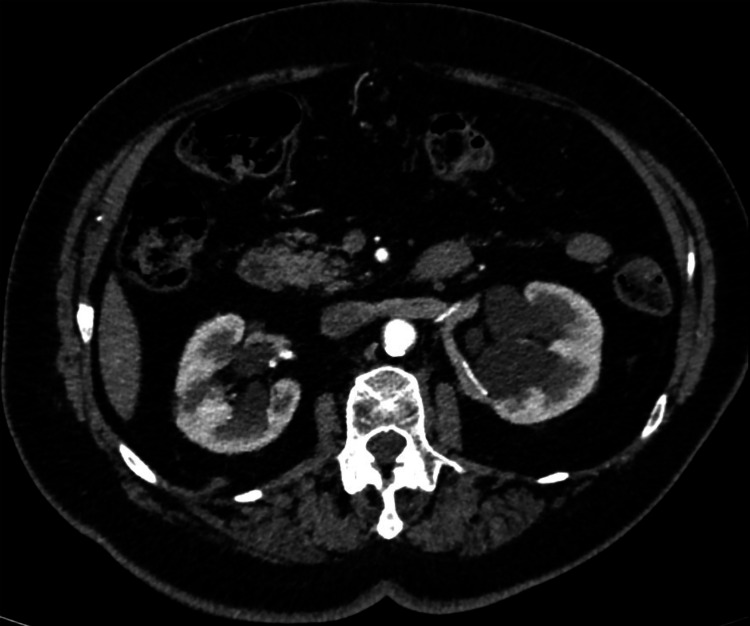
Axial cross section of an abdominal enhanced computed tomography (CT) scan showing bilateral non-enhancing multiple cortical and para-pelvic simple renal cysts. The largest is a para-pelvic left kidney cyst, measuring 5.3 cm in its greatest dimension. No calcifications or septations noted within those cysts.
